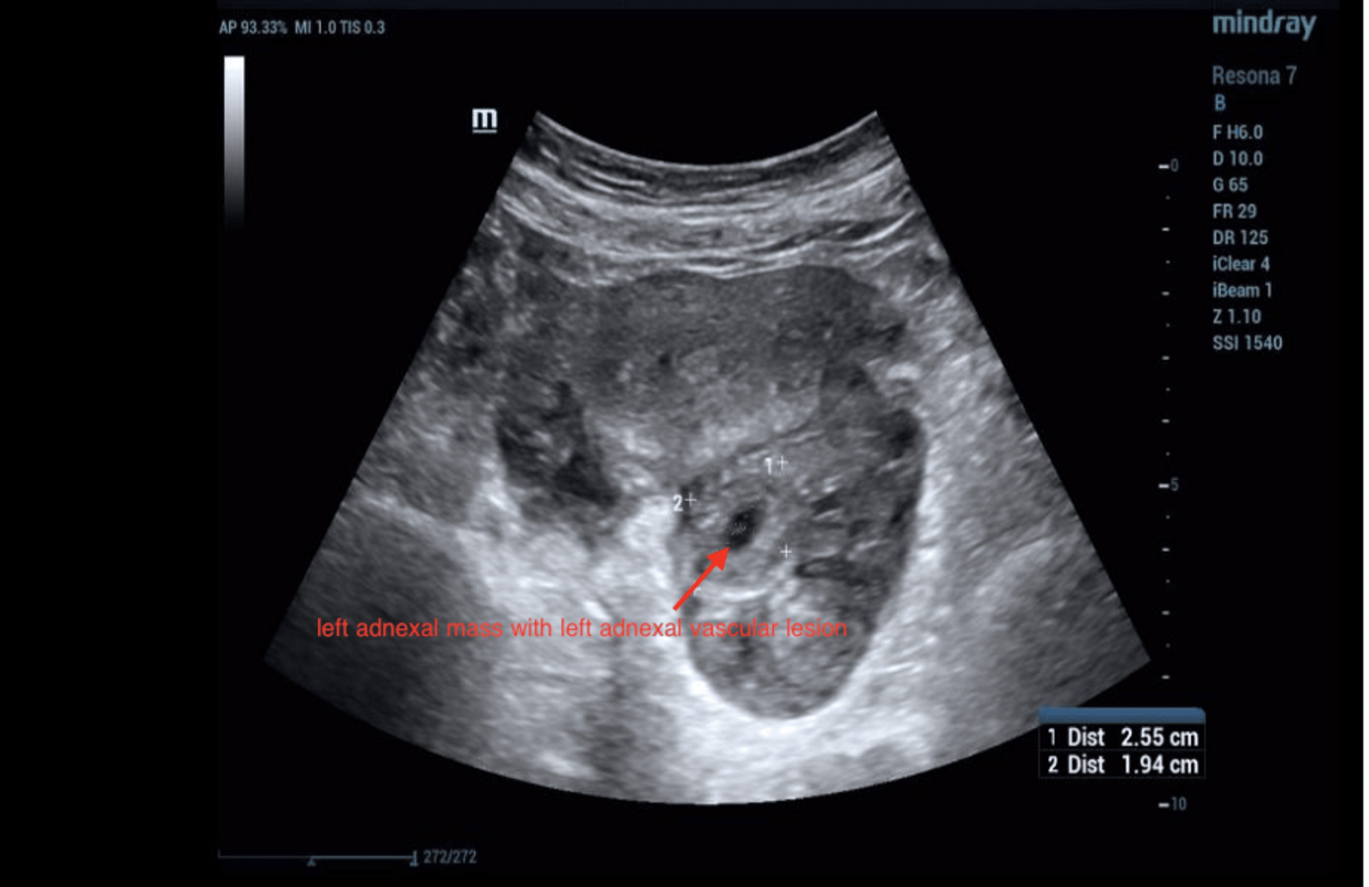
Transabdominal ultrasonography image of uterus shows a left adnexal mass with left adnexal vascular lesion (complicated left ectopic gestation).
